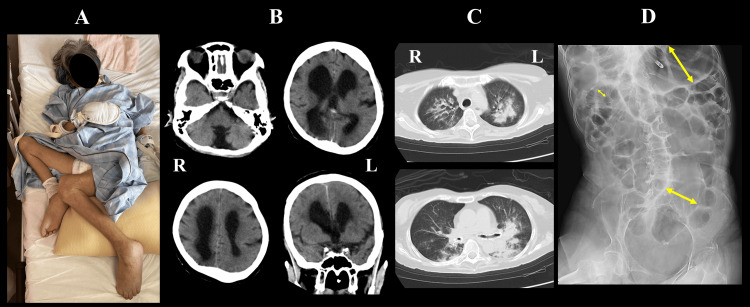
Patient’s condition on admission (A) Both hip and knee joints in the patient were contracted and fixed in a flexed position; (B) Brain CT shows aplasia of the cerebellar vermis and the presence of obviously enlarged ventricles; (C) Chest CT demonstrates evidence of pneumonia in the bilateral lung fields, predominantly in the left lung, with peribronchial and posterior localization suggestive of aspiration; (D) Abdominal X-ray reveals significant intestinal gas retention resembling ileus. The maximum diameters in the stomach, small intestine, and colon, where gas retention was observed, were measured on X-ray (yellow arrows).
